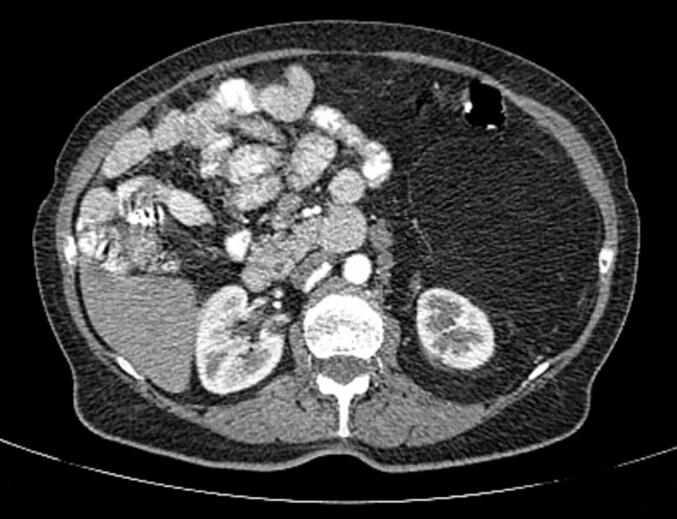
CT with both intravenous and oral contrast. Mass displacing the intestinal loops to the right and excluding the left colon anteriorly, with close relation to the Gerota's fascia and proximity to the left kidney.
